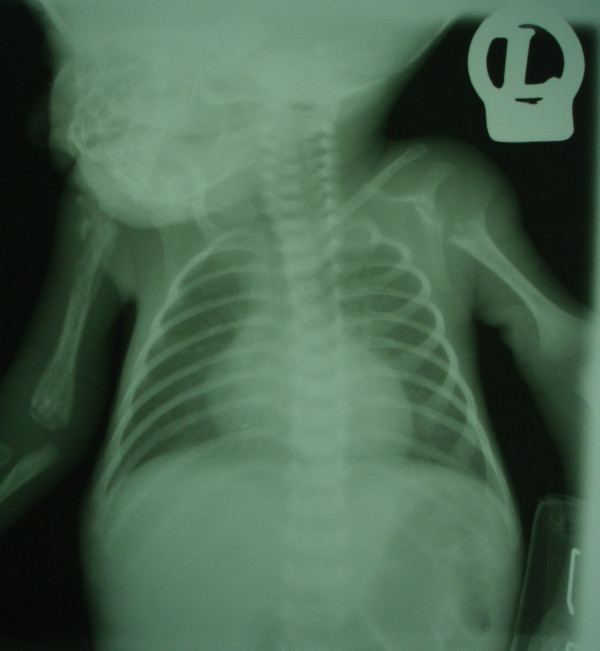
Chest X-ray obtained at the time of admission. Bilateral osteomyelitis in the humerus presented as osteolysis and involucrum. Also, there is sequestration because of osteonecrosis and a pathologic fracture in the proximal part of the humerus. Dislocation of right glenohumeral joint brings up the arthritis diagnosis. There is complete osteolysis in proximal metaphysis in the left humerus and arthritis in the left glenohumeral joint. The heart, lung and pleural space have a normal appearance, while the thymus is atrophic.
